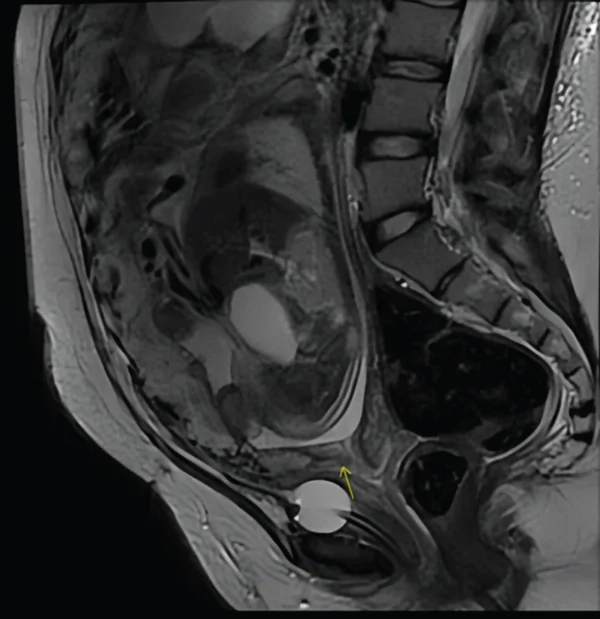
MRI appearance of the cervico‐isthmic region. Sagittal T2‐weighted MR image demonstrates cervical shortening and effacement with preservation of an identifiable endocervical canal free of gestational tissue.
